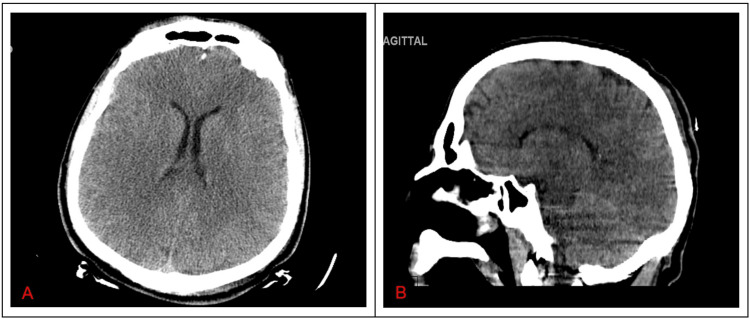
A CT of the head was negative for acute changes (A and B)
